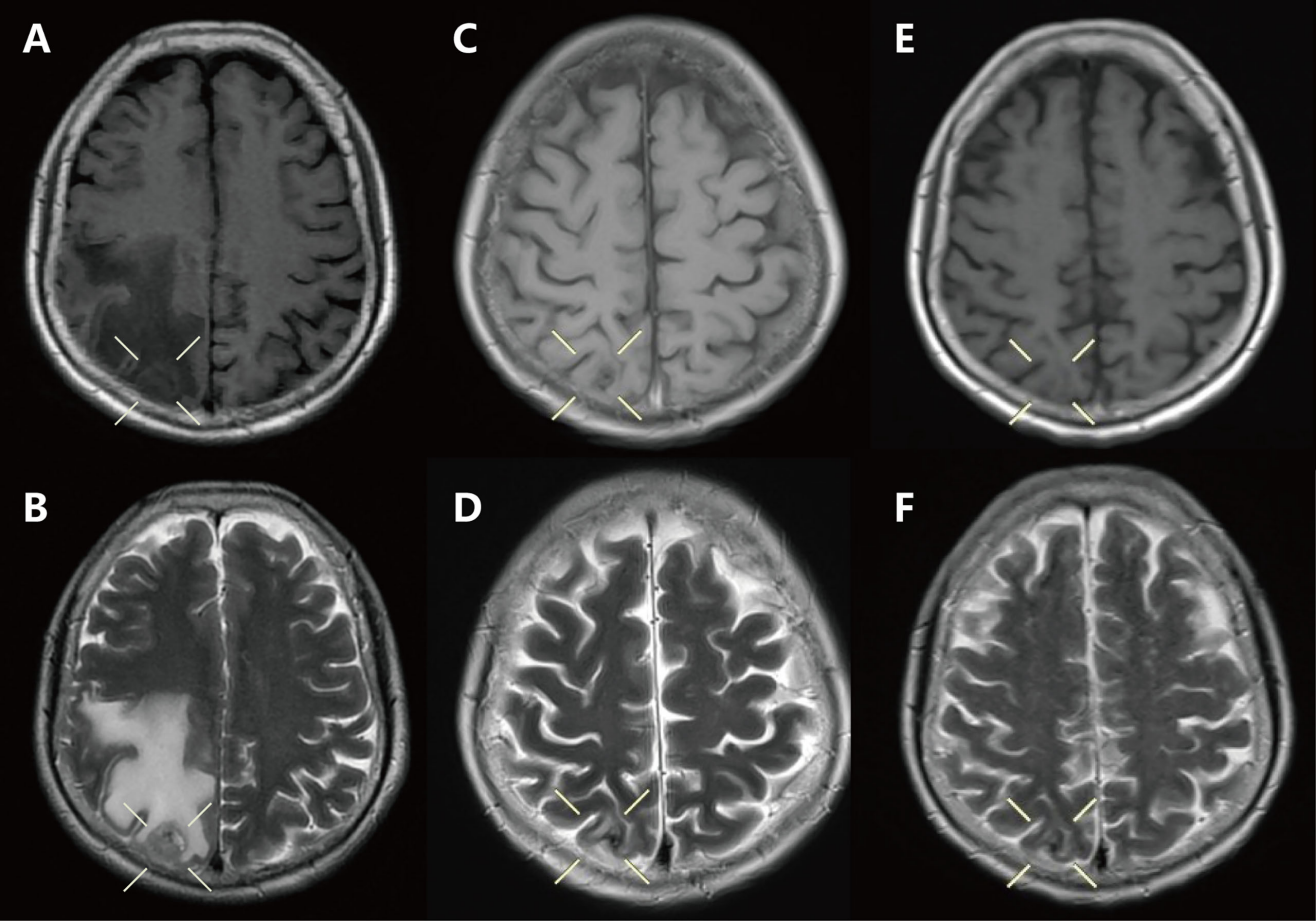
Craniocerebral magnetic resonance imaging of the brain showed: (A, B) Baseline: craniocerebral lesion before treatment. (C, D) Two months: after brain radiotherapy combined with osimertinib. (E, F) Seven months: after the use of erlotinib combined with capmatinib.
